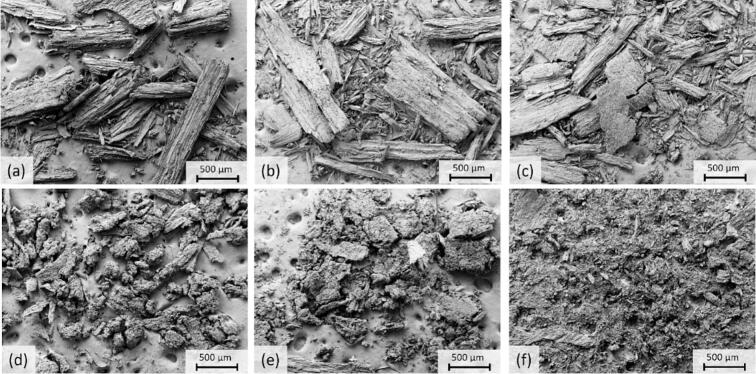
FESEM images (100x magnification) of sawdust (2.5 g) treated without ultrasound using (a) 1 %, (b) 2.5 %, and (c) 5 % formic acid and with ultrasound (70:30 pulse, 3 bar, 150–180 °C), using (d) 1 %, (e) 2.5 %, and (f) 5 % formic acid with 30 min extended reaction time in all treatments.
